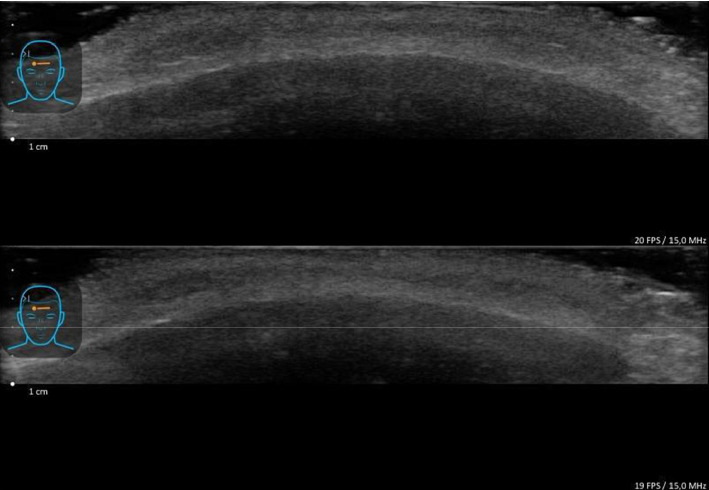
Ultrasound monitoring (15 MHz) of nodule resolution on Patient 1. Both images demonstrate intact anatomical layers with preserved dermal–subdermal junction and normal muscle echotexture, showing absence of fluid collections, granulomatous changes, or other structural complications.
